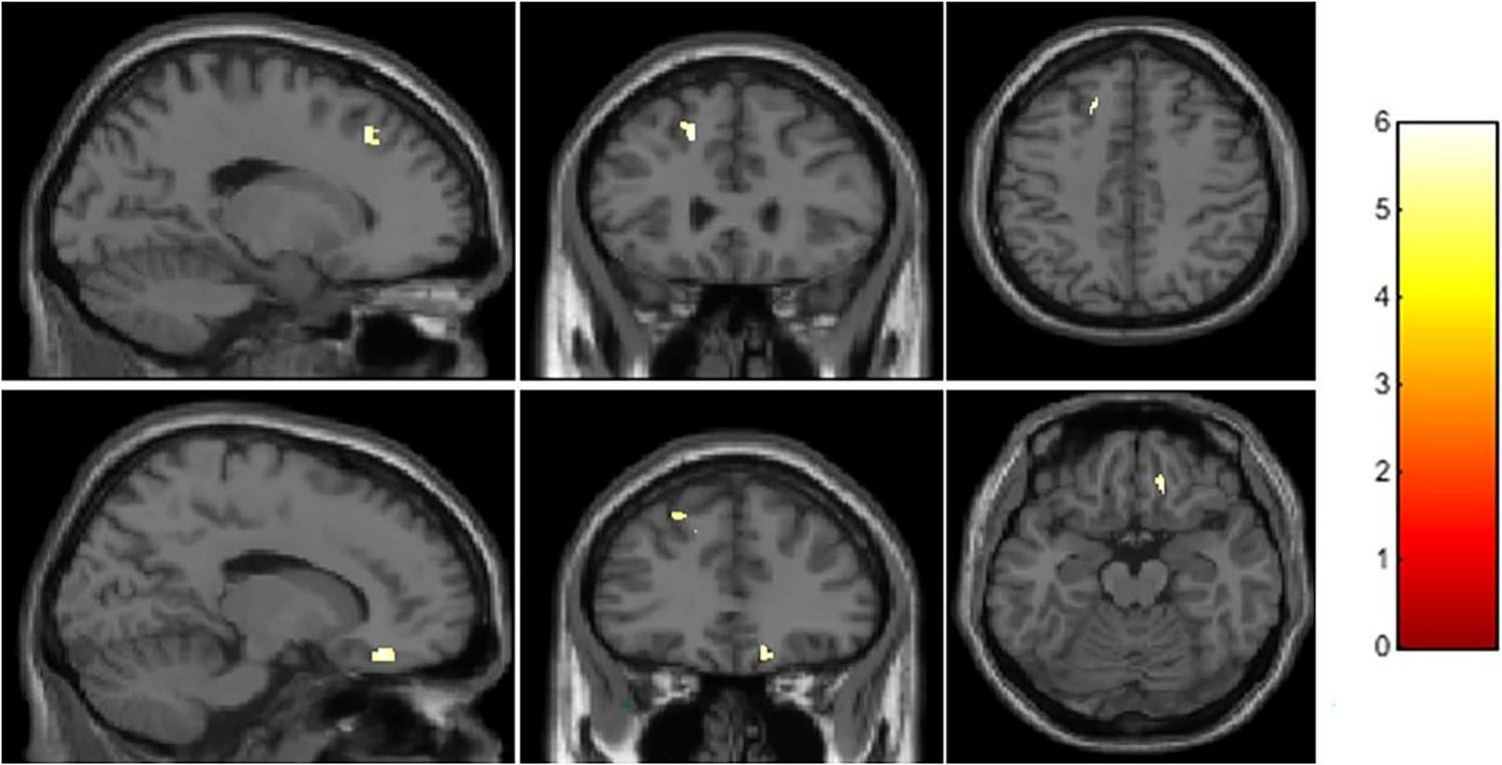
Statistical parametric maps overlaying averaged T1-weighted images show significantly smaller grey matter volume in the left superior and middle frontal gyrus (*x* = −15, *y* = 29, *z* = 42, cluster extent = 110) and right orbitofrontal cortex (*x* = 15, *y* = 35, *z* = −18, cluster extent = 54) in smokers compared with healthy non-smokers.
